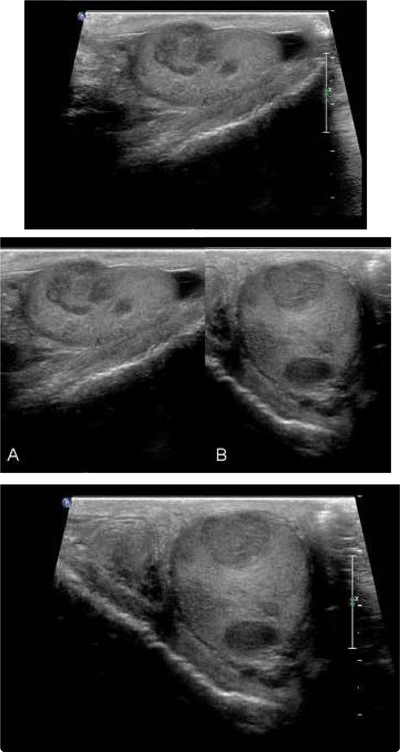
Scrotal ultrasound of left testis. Ultrasound reveals multiple hypoechoic masses within the left testicle, some abutting the tunica albuginea without frank extratesticular invasion. Shown in: A, longitudinal and B, transverse orientations.
